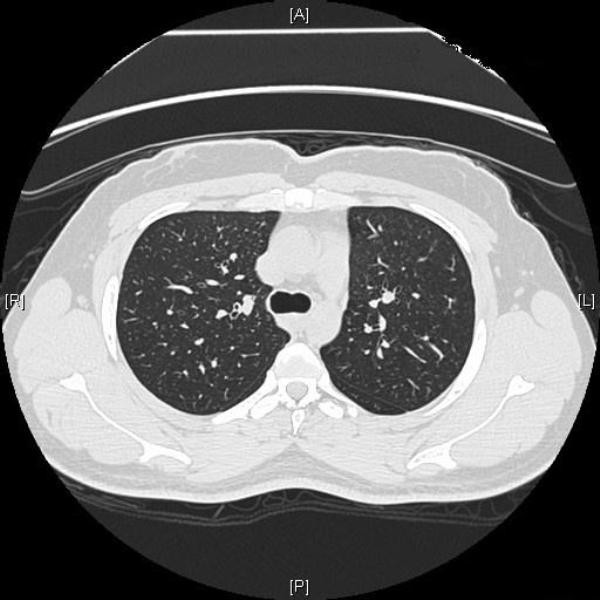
Case 4. HRCT scan obtained at the upper lung zones showing poorly defined centrilobular nodules with diffuse bronchial wall thickening throughout both lung fields.
